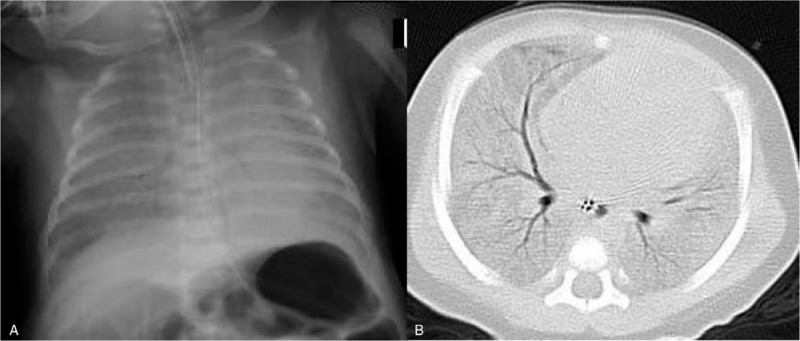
Case 2: (A) Chest radiograph showing widespread ground glass opacification of the bilateral lungs; (B) Computed tomography image showing consolidation with air bronchogram in both lungs.
